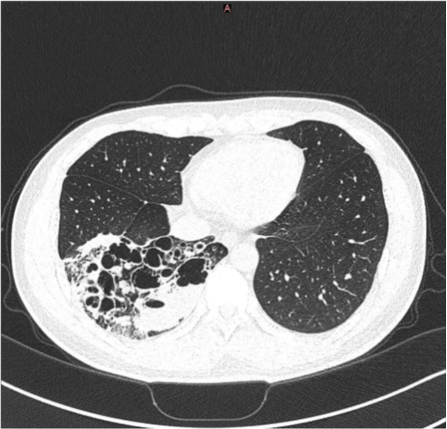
CT scan of the thorax (lung window) showed that the right lower lobe had been very extensively replaced by multi-cystic changes and diffuse inflammation. Other than sequestration, differential diagnoses at that time included CCAM and bronchiectasis
